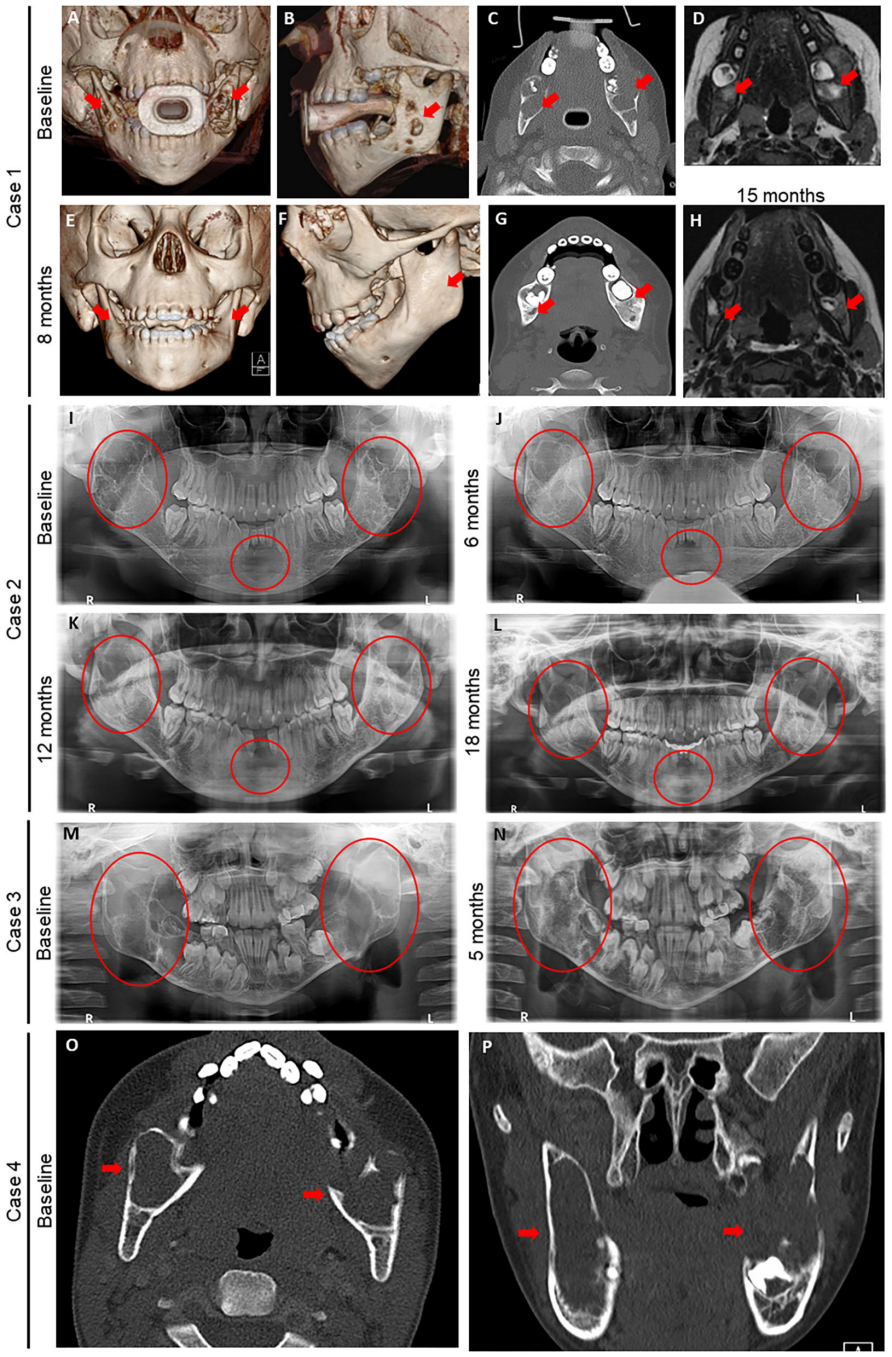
Radiography before and after denosumab in four children with Noonan-like/multiple giant cell lesions (NL/MGCLs). (A–H) Case 1 baseline images (A–D) of 3-dimensional (3D) computed tomography (CT) reconstruction of frontal (A) and profile (B) views, axial CT without contrast (C), and axial magnetic resonance imaging (MRI) (D) at the level of mandibular molars relative to post-denosumab (E–H) 3D CT reconstruction of frontal (E) and profile (F) views, axial CT without contrast (G) 8 months after initial denosumab treatment, and axial magnetic resonance imaging (MRI) (F) at the level of mandibular molars 15 months after second denosumab treatment. (I–L) Case 2 dental orthopantomogram at baseline (I) and after on denosumab for 6 (J), 12 (K), and 18 (L) months. (M, N) Case 3: dental orthopantomogram at baseline (M) and after on denosumab for 5 months (N). (O,P) Case 4: baseline axial (O) and coronal (P) CT without contrast at level of mandibular molars. Red arrows and circles denote NL/MGCLs.
